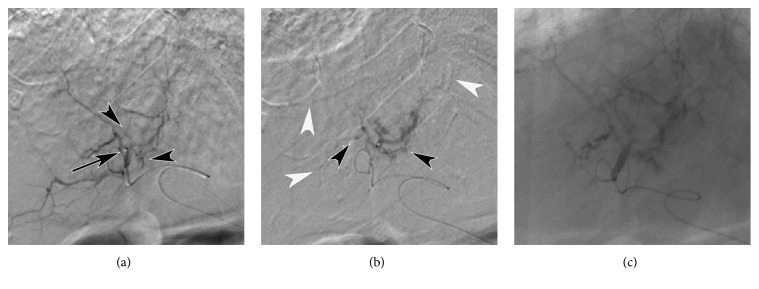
Arterial occlusion process of RAIB-TACE (same case of Figure 4). (a) DSA before RAIB-TACE showing a microballoon catheter at the superior posterior subsegmental artery (black arrow) and multiple small tumor feeders branching from proximal portion (black arrowheads). (b) DSA obtained after 2 repetitions of alternate infusion showing earlier embolization of distal branches (white arrowheads) and patent proximal tumor feeders (black arrowheads). (c) Spot radiography immediately after RAIB-TACE (5 repetitions of alternate infusion) showing stasis of contrast medium in both the distal branches and proximal tumor feeders.
